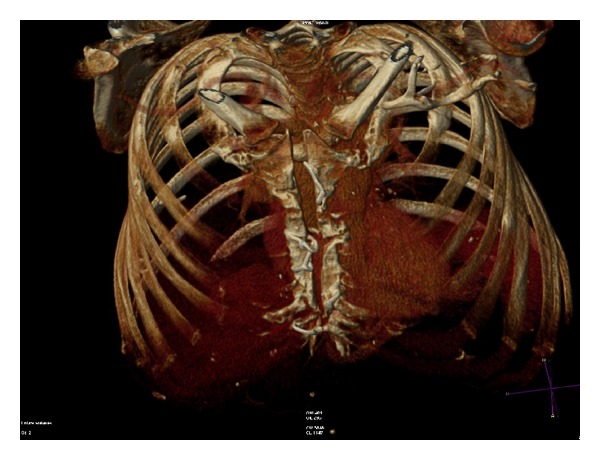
CT reconstruction of sternal dehiscence.
